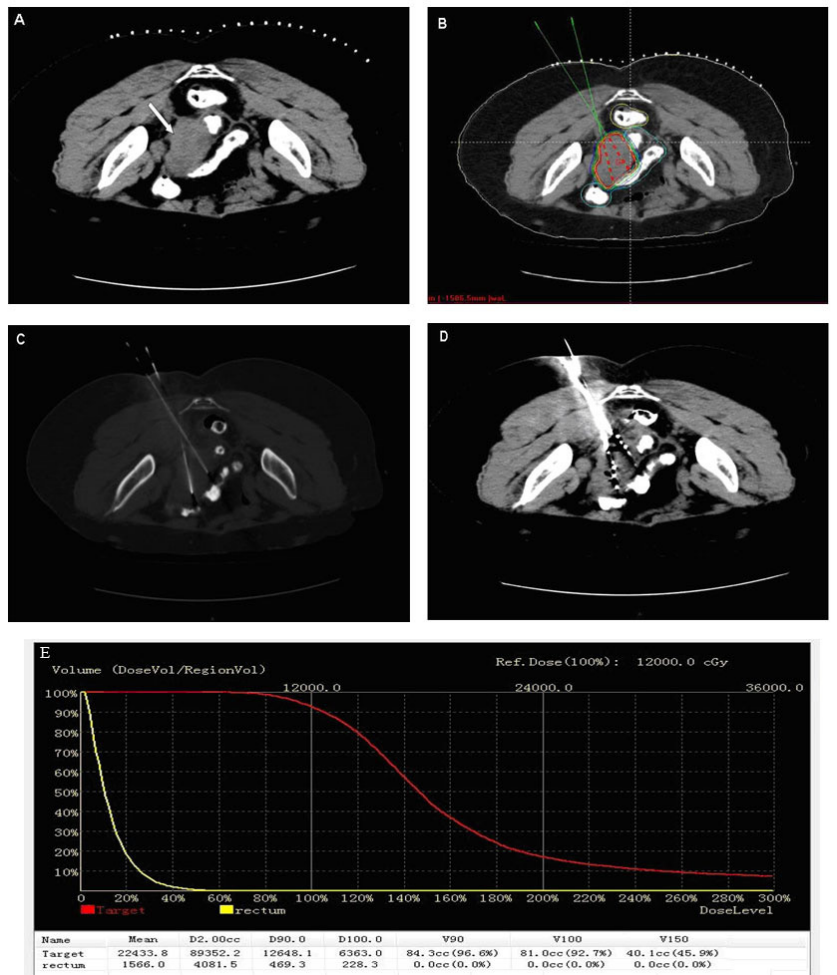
The administration of ^125^I seeds brachytherapy. A. Preoperative transverse CT image was obtained for targeting area of interest. B. The isodose curve on treatment planning system. The position of brachytherapy applicator and the dose were calculated by treatment planning system. C. The implantation of applicators. D. The administration of ^125^I seeds. E. A dose-volume histogram of PTV and risk organ after seeds implantation.
